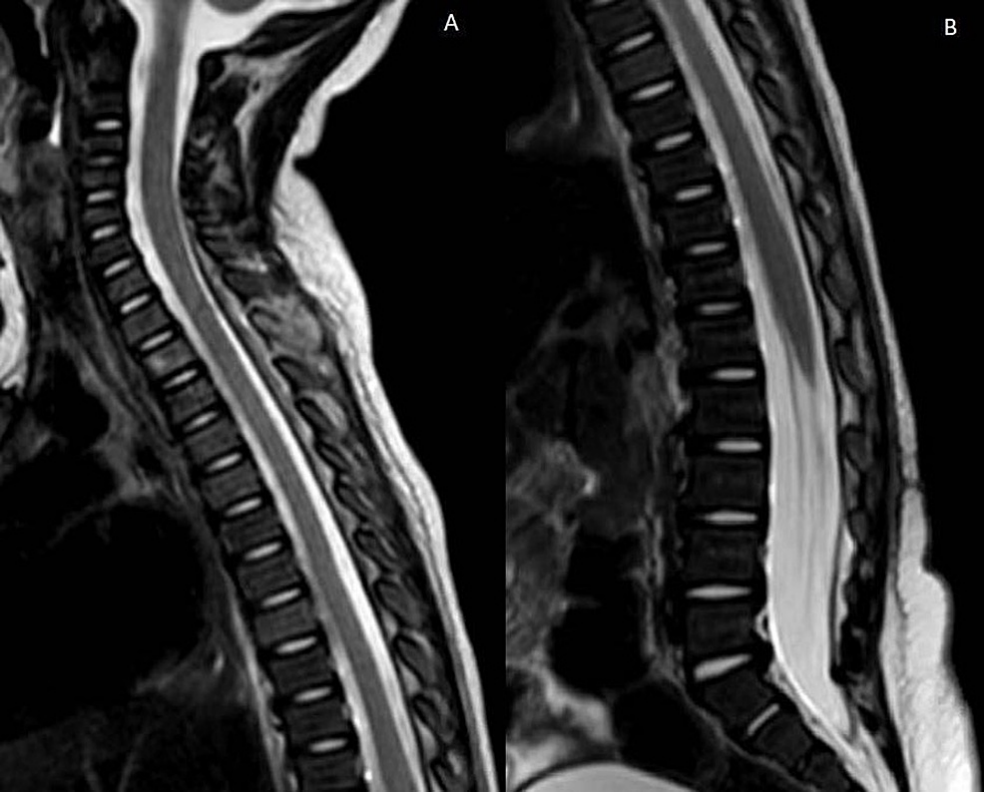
Three-week postoperative T2 MRI of the cervicothoracic (A) and thoracolumbar (B) regions demonstrate near-complete resolution of the spinal epidural abscess. MRI: magnetic resonance imaging
